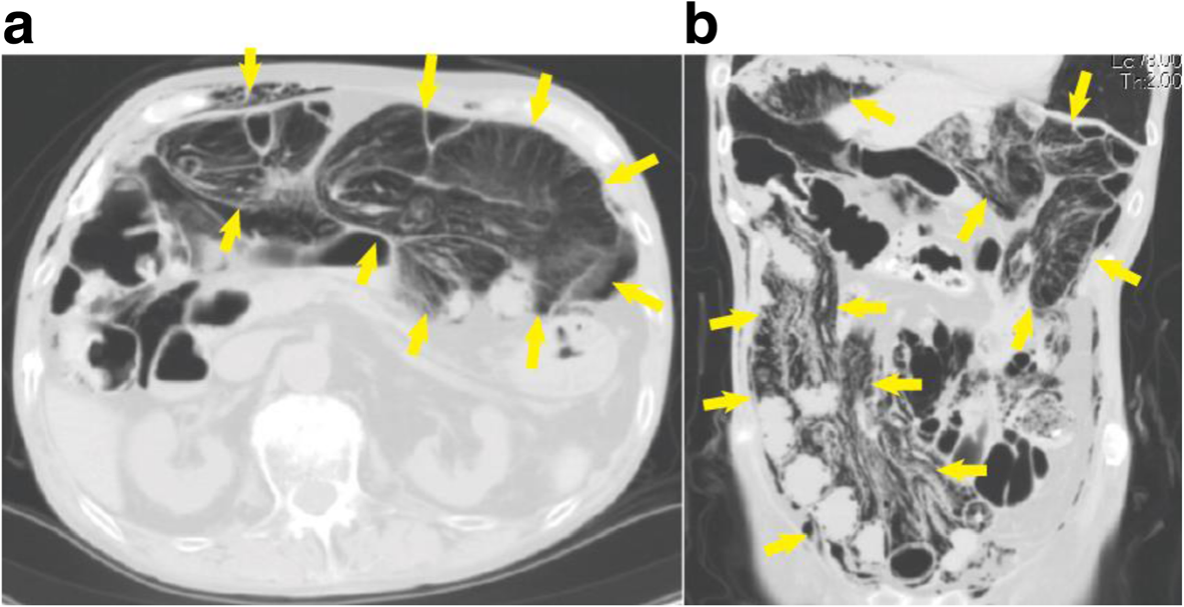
Computed tomography shows a large amount of gas in the abdominal wall, bowel wall, and mesentery (*arrows* in axial view (a) and in coronal view (b)). It was difficult, however, to rule out the existence of free air in the abdominal cavity. Portomesenteric pneumatosis was not detected
